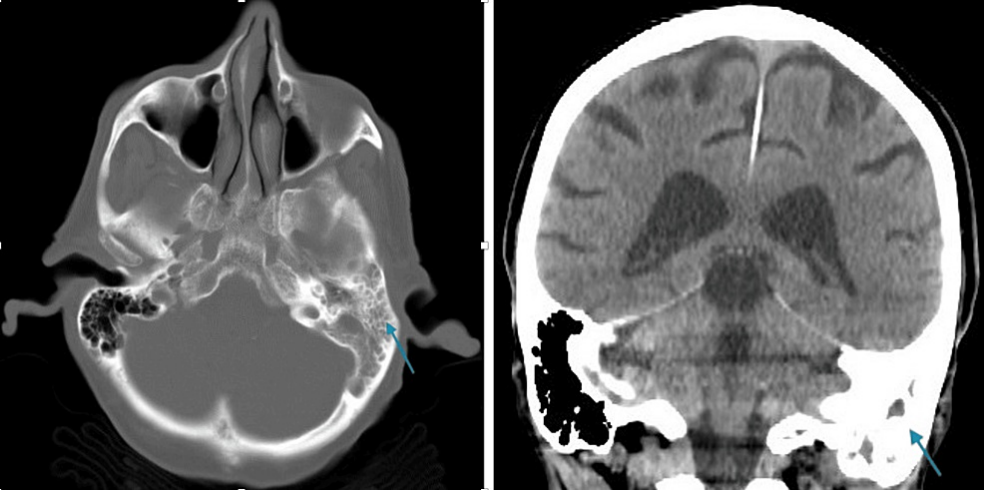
Noncontrast CT scan axial (left panel) and coronal (right panel) views demonstrating significant opacification of the mastoid air cells (blue arrow).
